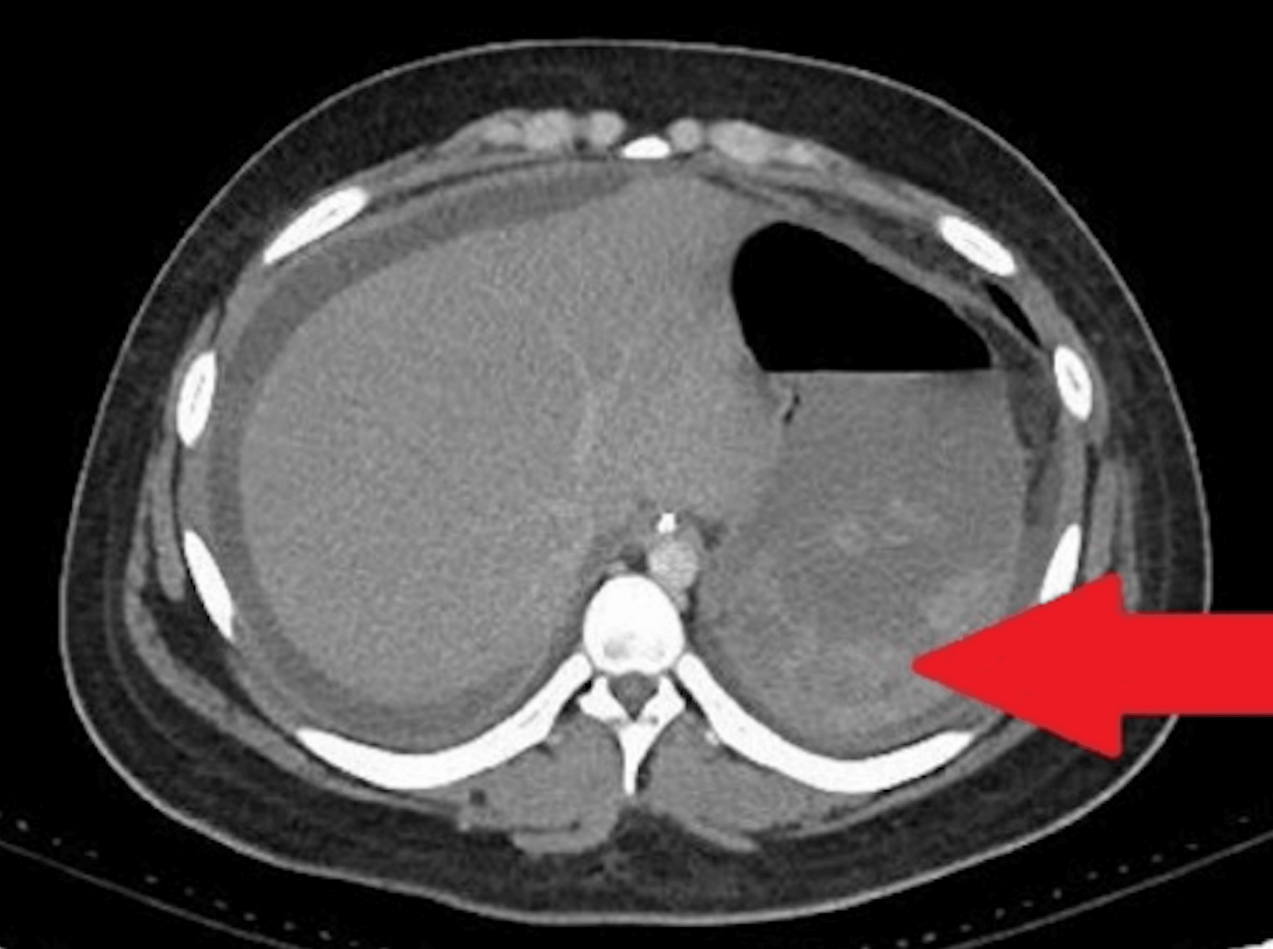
CT abdomen showing mixed echogenic debris in the gastric lumen. CT, computed tomography.
